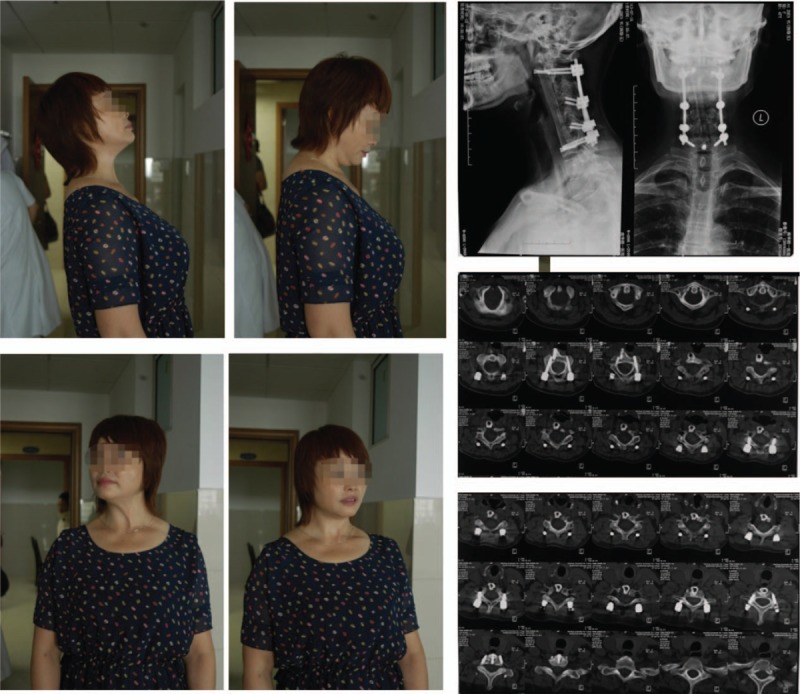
Scans at 18 months postsurgery showed satisfactory recovery of cervical function. Patient performed anteflexion, rear protraction, and lateral neck bending. X-ray and CT scans demonstrated no recurrence of TB and the internal fixation and fusion were excellent. CT = computed tomography, TB = tuberculosis.
